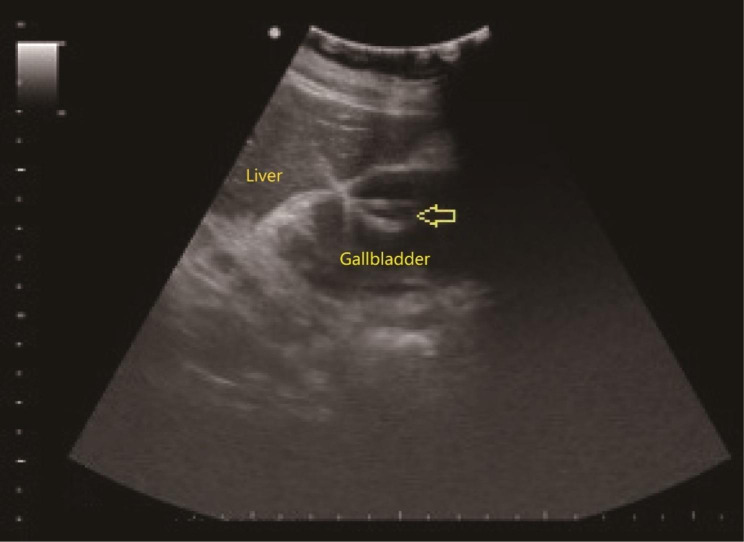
Image of ultrasound guided percutaneous transhepatic gallbladder drainage. An 8 F pigtail drainage catheter was placed in the gallbladder cavity (arrow)
